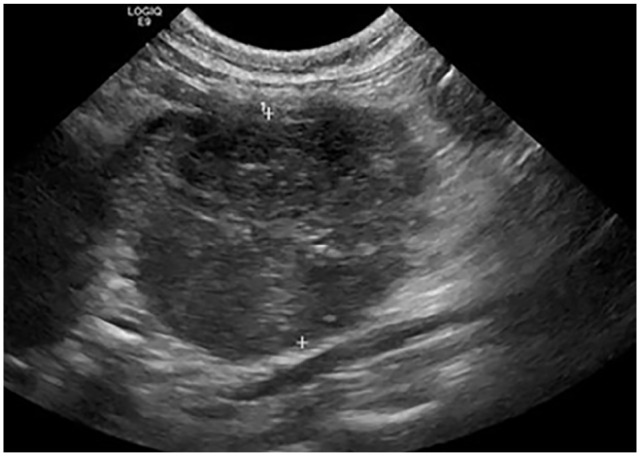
Abdominal ultrasound image of an enlarged mesenteric lymph node, measuring
